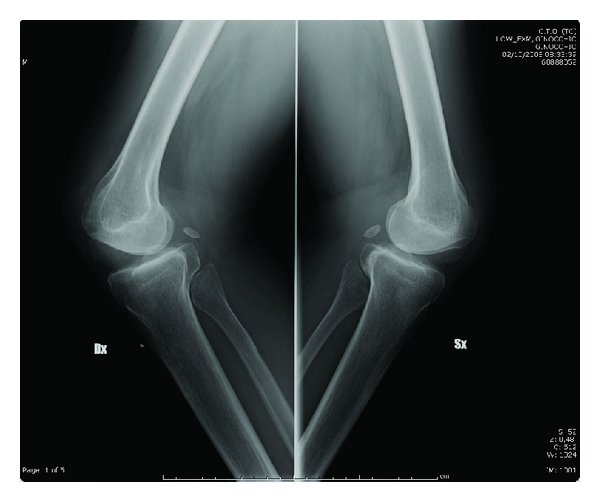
Lateral roentgenograms of the knees at about 30 degrees of flexion, showing dislocated patellae, marked lateral rotation deformities of the tibiae, and condylar deformity.
